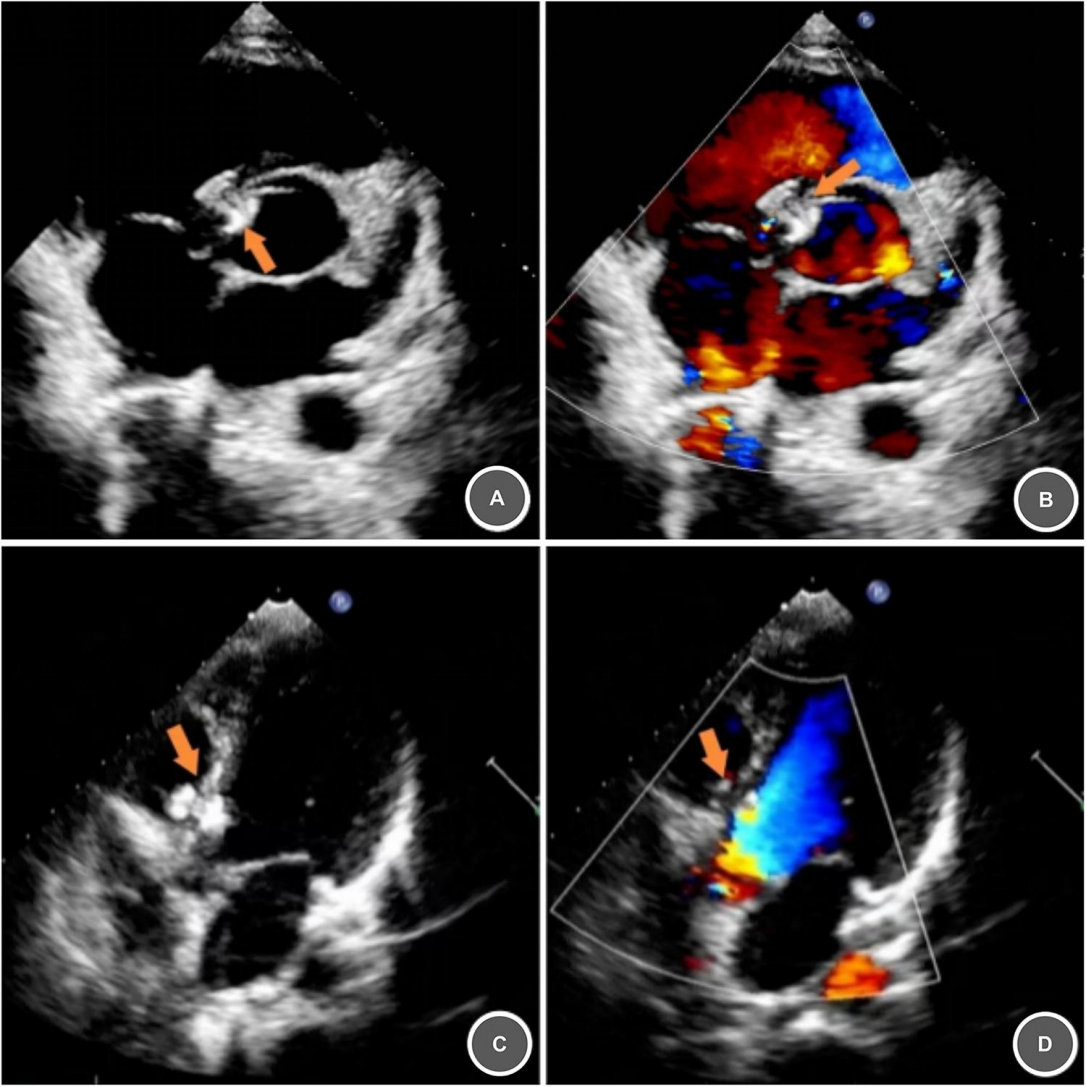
Immediate and one–month postoperative echocardiographic follow, up after VSD closure. (A,B) Postoperative echocardiography demonstrating a well-positioned and stable occluder with no significant residual shunt (arrow). (C,D) One-month postoperative echocardiography shows the occluder is well-positioned and stable, with no significant residual shunt (arrow).
